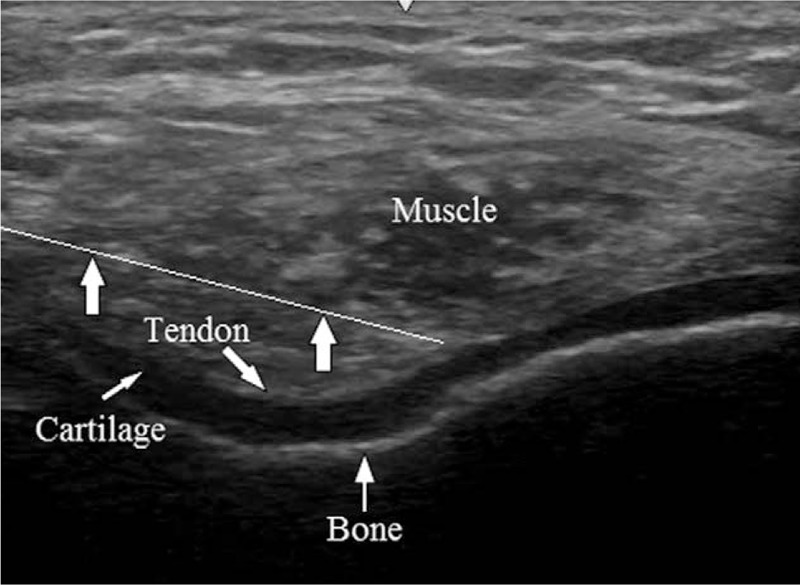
Ultrasound image during injection of polynucleotides in the knee joint space (arrow indicates the block needle.).
